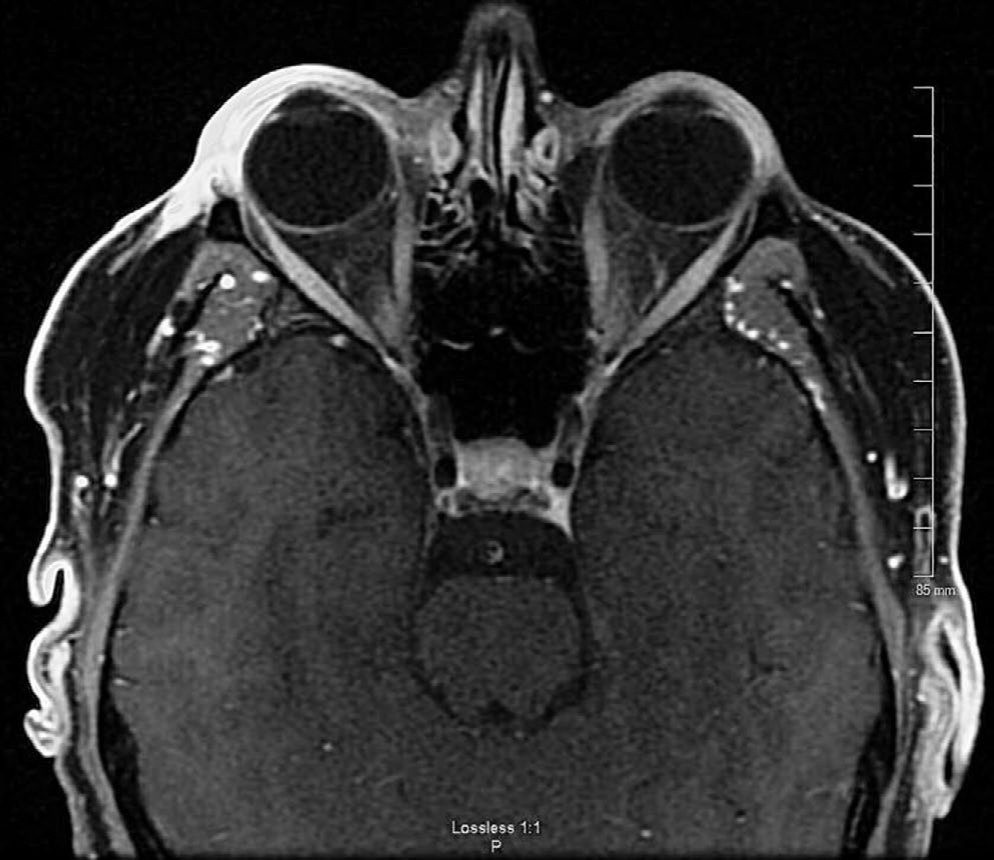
Magnetic resonance imaging (MRI) of the brain with contrast. Right eye showing significant subcutaneous tissue edema and enhancement. Postseptal area, eyeball, and optic nerve appear within normal limits
